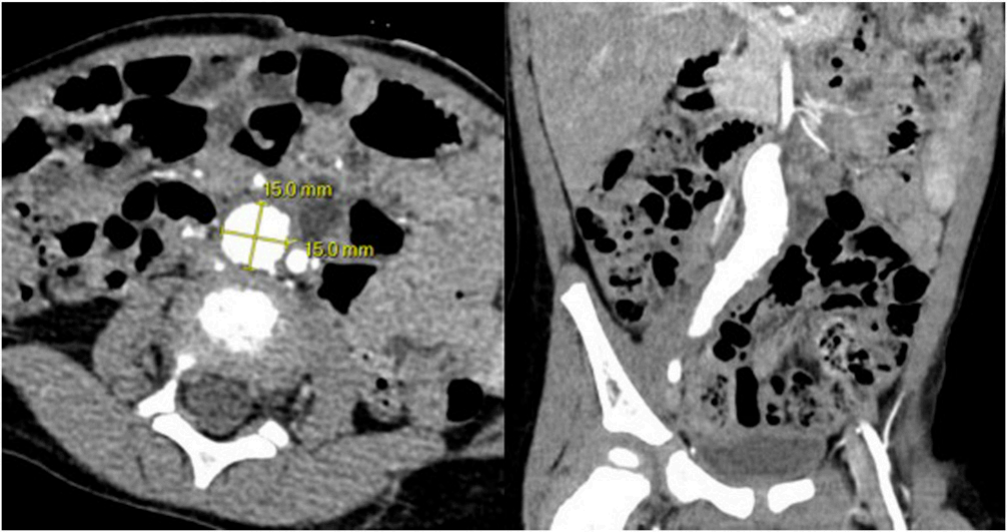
Axial and coronal CT sections demonstrating a 1.5 × 1.5 cm right common iliac artery aneurysm.
